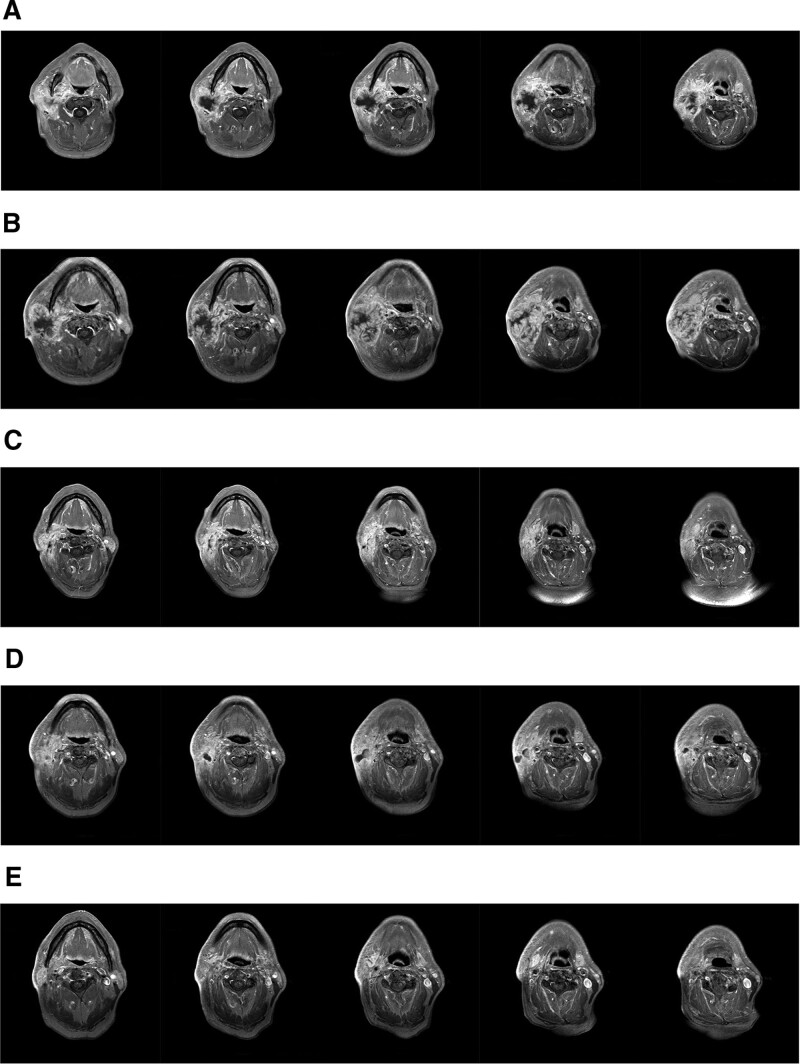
A: Head and neck MRI revealed a recurrence (January 29, 2021). B: MRI showed that the mass was larger than the previous (February 19, 2021). C: MRI revealed that the metastasis was significantly receded (March 16, 2021). D: MRI detected that the tumor has shrunk by 80% compared to the previous (April 8, 2021). E: Head and neck MRI revealed CR (May 24, 2021). CR = complete response, MRI = magnetic resonance imaging.
